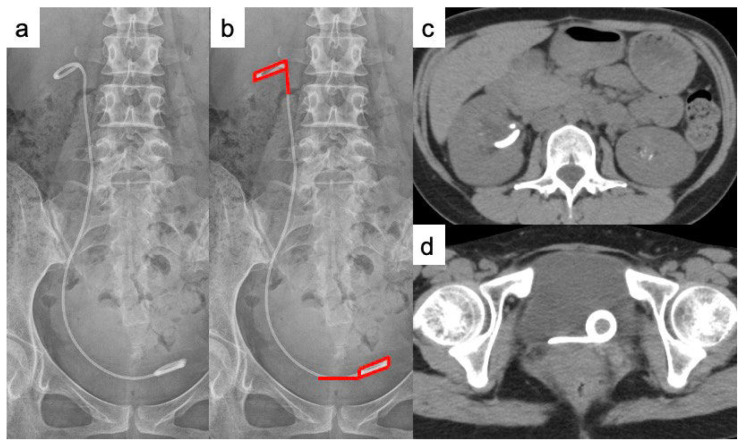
Findings of KUB and CT 13 weeks after placement of a ureteral stent a. Ureteral stent encrustation is not detected by X-ray imaging. b. The red lines show location of ureteral stent encrustation. c. The proximal coil is thick for a ureteral stent. d. The distal coil is thick for a ureteral stent.
